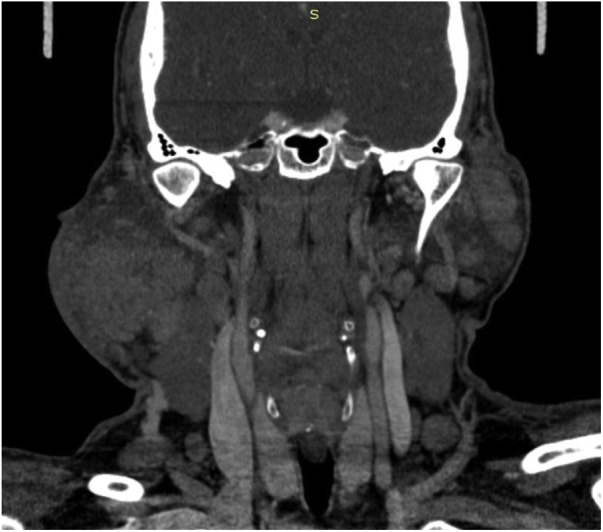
Computed tomography of the neck (coronal view) showing bilateral diffuse enlargement of the parotid glands, right more than left, multiple nodules of varying sizes in the superficial and deep lobe of both glands. Both the internal jugular veins and carotid vessels are preserved.
